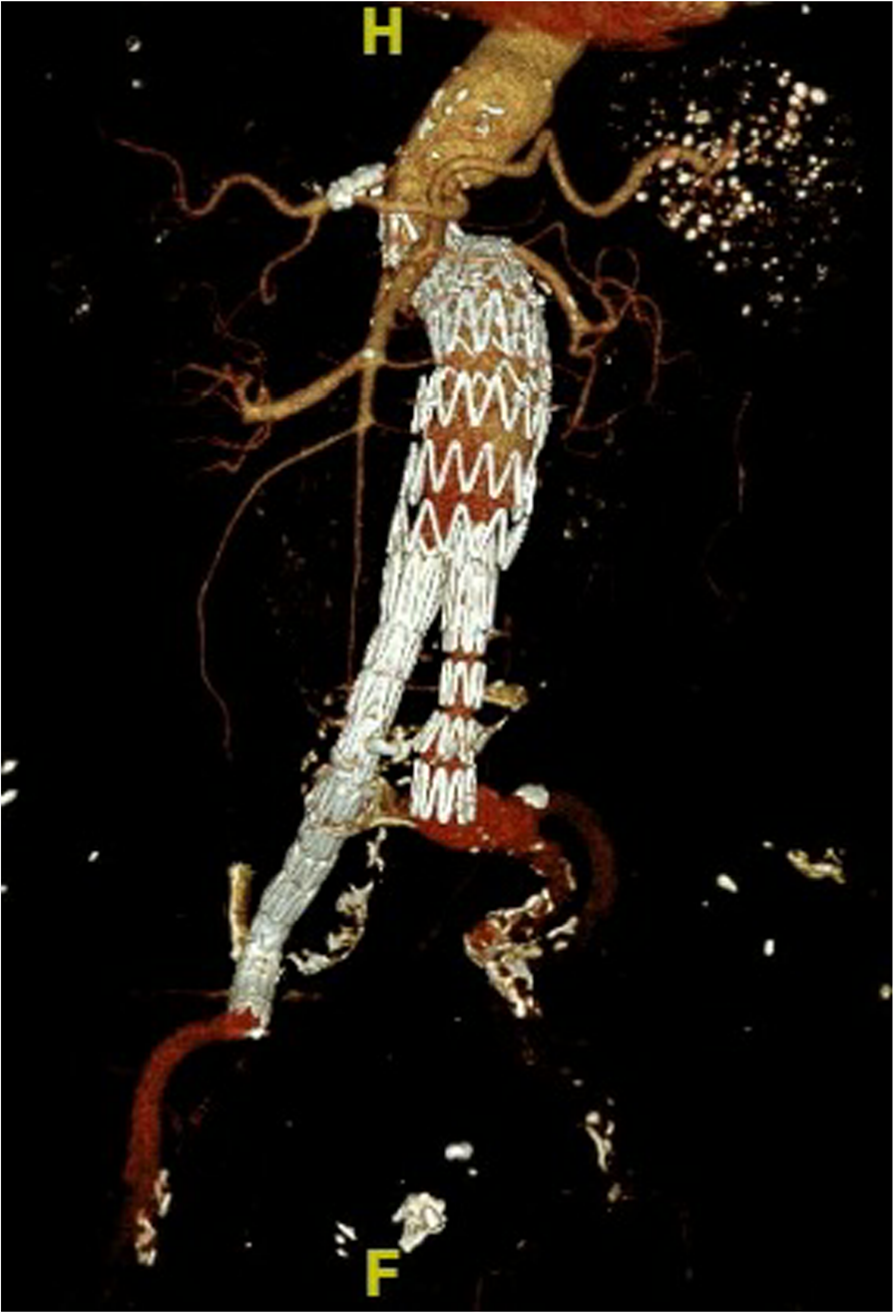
CTA was taken after the surgery but before discharging from hospital. Endoleak has disappeared
